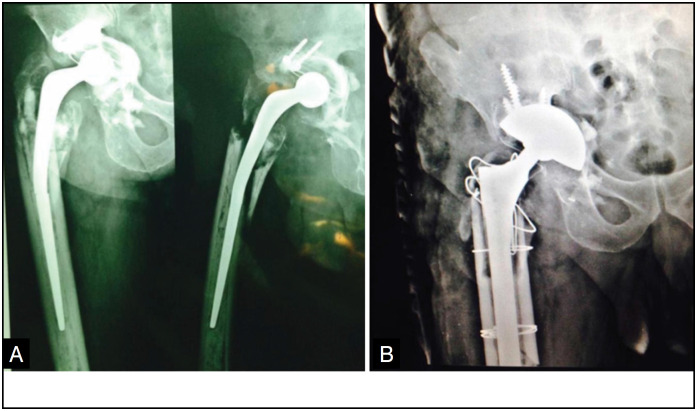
Case 02 (Image A: Preoperative X-ray and image B: X-ray six months after surgery).
